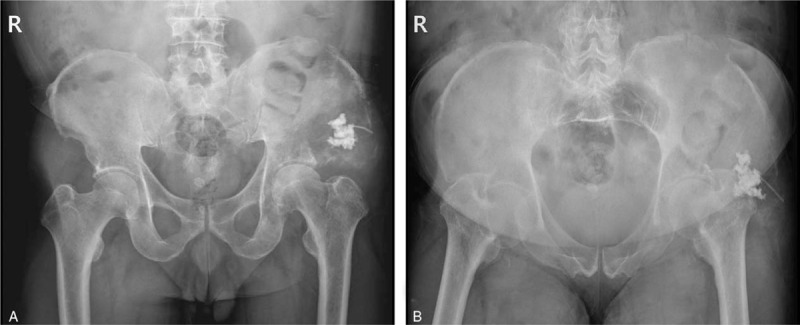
Post-procedure pelvic radiographs. Anteroposterior view (A) and oblique view (B).
